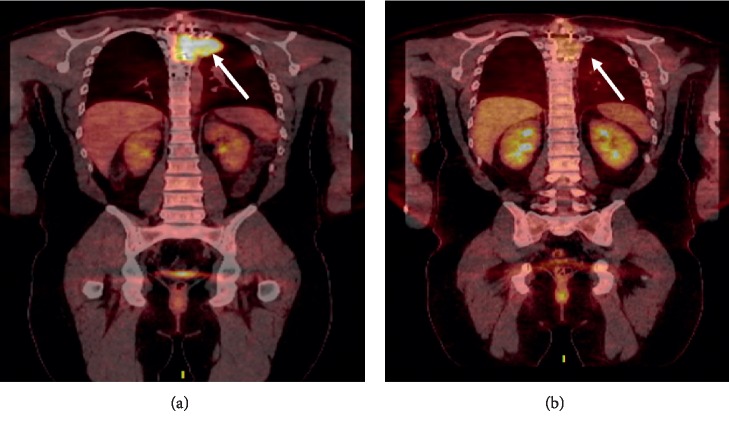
(a) 18F FDG PET-CT at relapse showing large soft-tissue mass replacing the T4 vertebral body (white arrow). (b) 18F FDG PET-CT after 2 cycles of KRD-PACE showing near-complete resolution of the extraosseous soft-tissue component involving patient's known T4 vertebral body lesion (white arrow).
